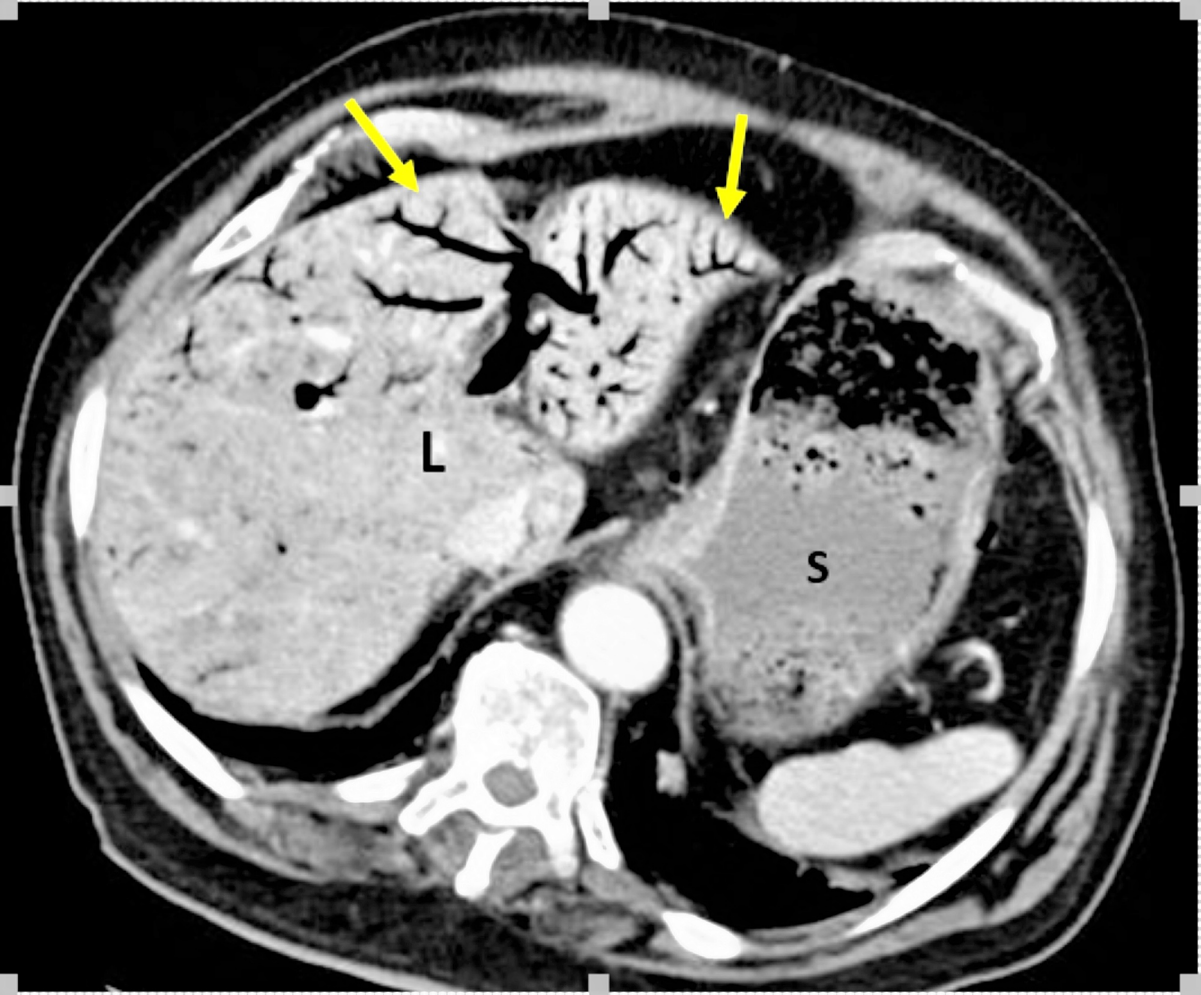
Contrast-enhanced CT scan of the Abdomen, axial view, L shows liver with yellow arrows pointing to the branching pattern of portal venous gas. S shows a dilated stomach with food contents
